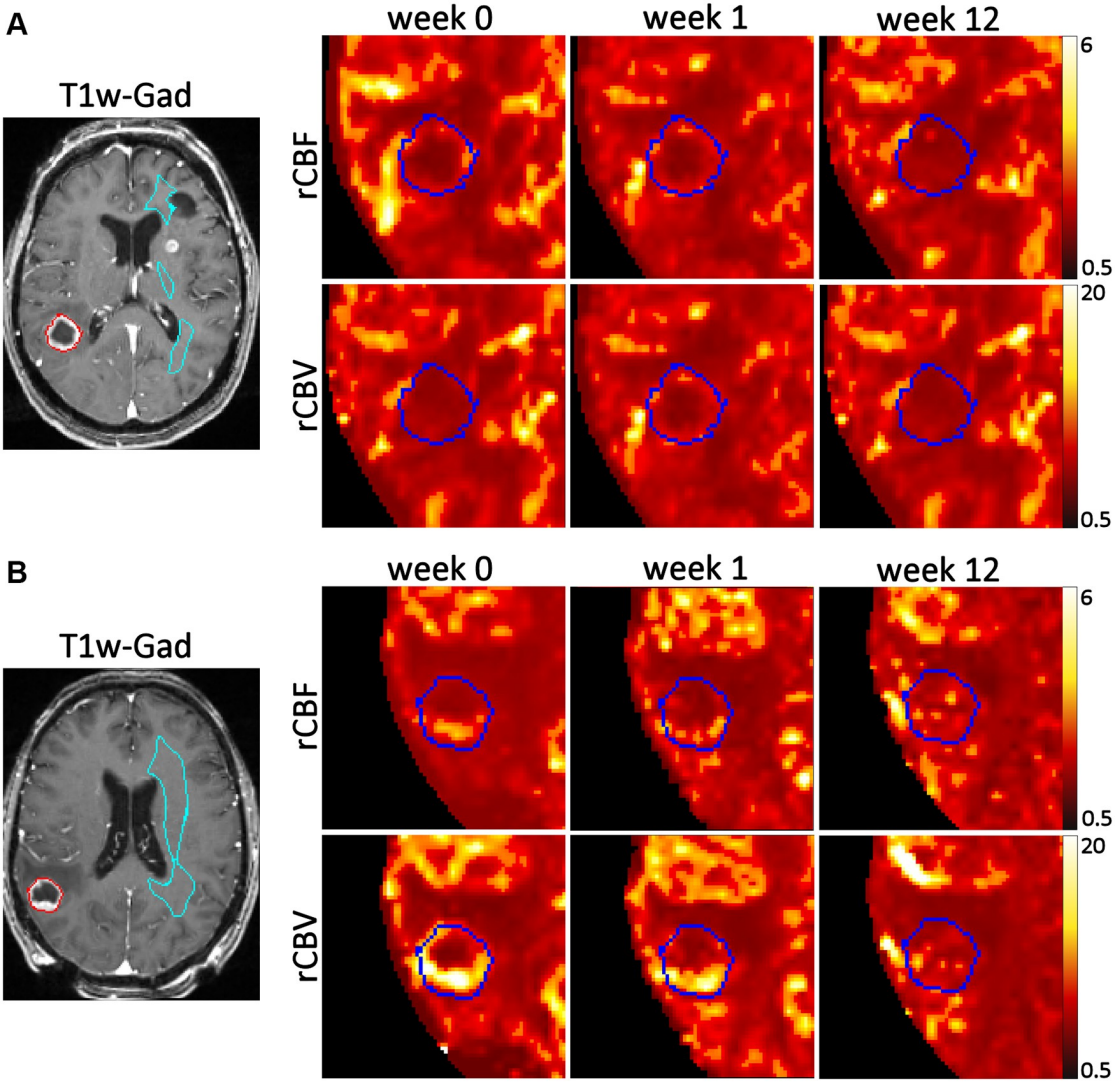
Representative axial MRI and rCBF and rCBV maps before and after radiosurgery. A) A lesion that did not develop local recurrence. B) A lesion that developed local recurrence. Red/dark blue outlines the treated lesion, cyan blue outlines the contralateral white matter normalization region. T1w-Gad represents post-contrast T1-weighed MRI sequence before radiosurgery.
